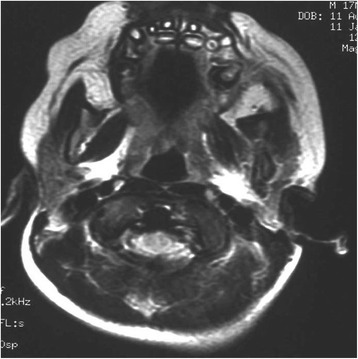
Magnetic resonance imaging performed after chemotherapy, showing no sign of soft tissue involvement with complete regression of the disease
